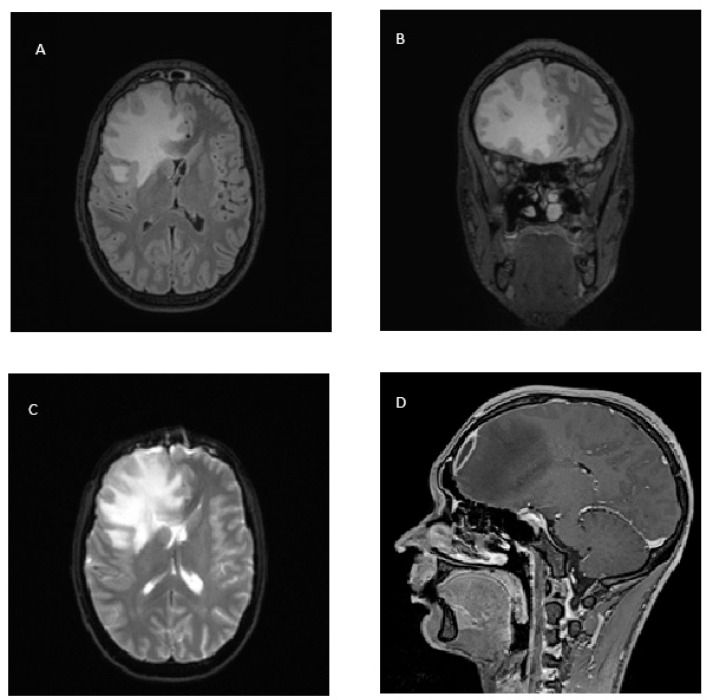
Magnetic resonance of the right frontal lobe showing cerebritis and abscess with ring enhancement and midline shift. (A,B) Axial and coronal fluid attenuated inversion recovery (FLAIR) images show an extensive hyperintense lesion in the right frontal lobe, with surrounding vasogenic edema and mass effect, consistent with cerebritis and abscess formation. (C) Axial diffusion-weighted imaging (DWI) images demonstrate marked hyperintensity within the lesion, indicative of restricted diffusion, characteristic of a pyogenic abscess. (D) Post-contrast T1-weighted image with fat saturation (sagittal view) reveals peripheral ring enhancement with central hypointensity, confirming the encapsulated nature of the abscess. Note the associated compression of the right lateral ventricle and midline shift.
